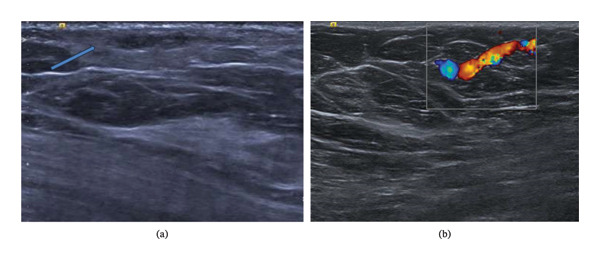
(a and b): A 70‐year‐old patient with a history of left breast carcinoma reported mastodynia at the junction of the upper quadrants of the right breast. The ultrasound shows an anechoic area with a cord‐like morphology in the superficial area (arrow in a) corresponding to a palpable nodule without internal flow on Colour Doppler imaging, consistent with a thrombosed vein. In b, the same vein after resolution of the thrombosis: there is a colour signal in the vessel on the Colour Doppler module.
